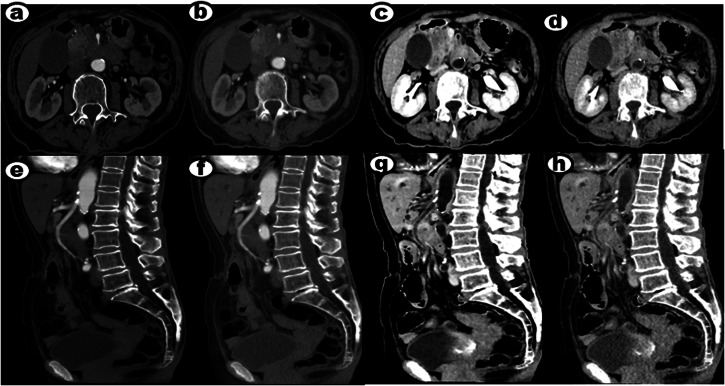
An 83-year-old woman was diagnosed with PDAC involving the head of the pancreas, with invasion of the SMA. a–d Axial arterial phase images were reconstructed using AiCE, AIDR 3D, AiCE–BBCT, and AIDR 3D–BBCT algorithms, providing detailed visualization of the tumor and the invaded SMA. The images demonstrated the presence of the tumor and the extent of vascular invasion. e–h Sagittal MPR CT angiographies were reconstructed at the same window and center levels using AiCE, AIDR 3D, AiCE–BBCT, and AIDR 3D–BBCT algorithms. These images further highlighted the invaded SMA, clearly delineating its involvement with the tumor. The slice thickness for image reconstruction was 1 mm. The BBCT technique effectively suppressed the enhanced CT value within the vascular lumen, resulting in improved visualization of the vascular wall. This allowed for a more accurate assessment of the invasion of the SMA by the PDAC
